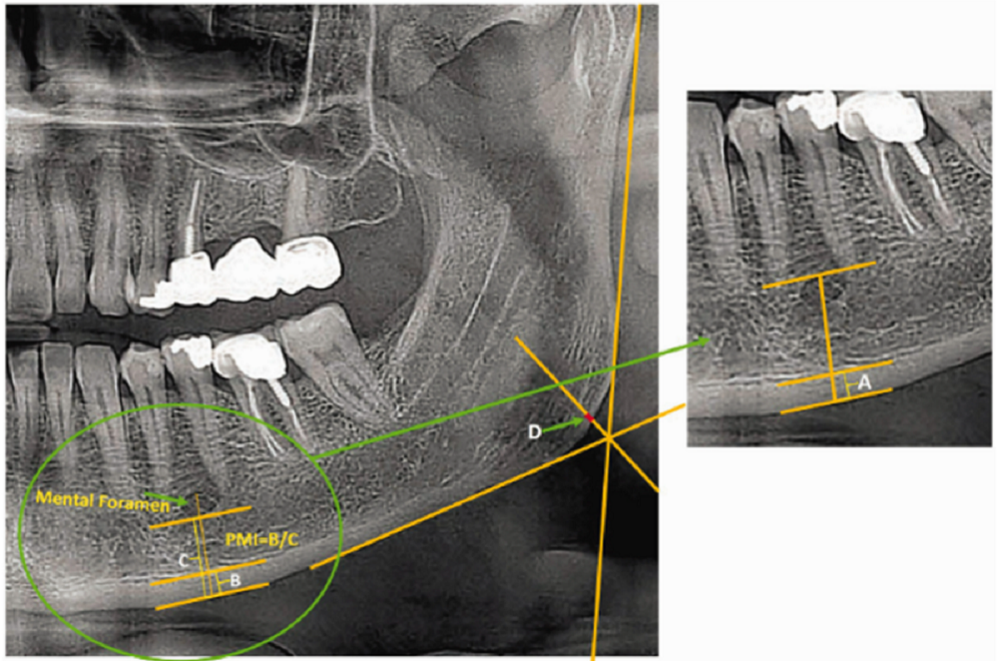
Graphic and radiographic representation for calculations of the panoramic mandibular index (width B/width C) and gonial index (width D).
